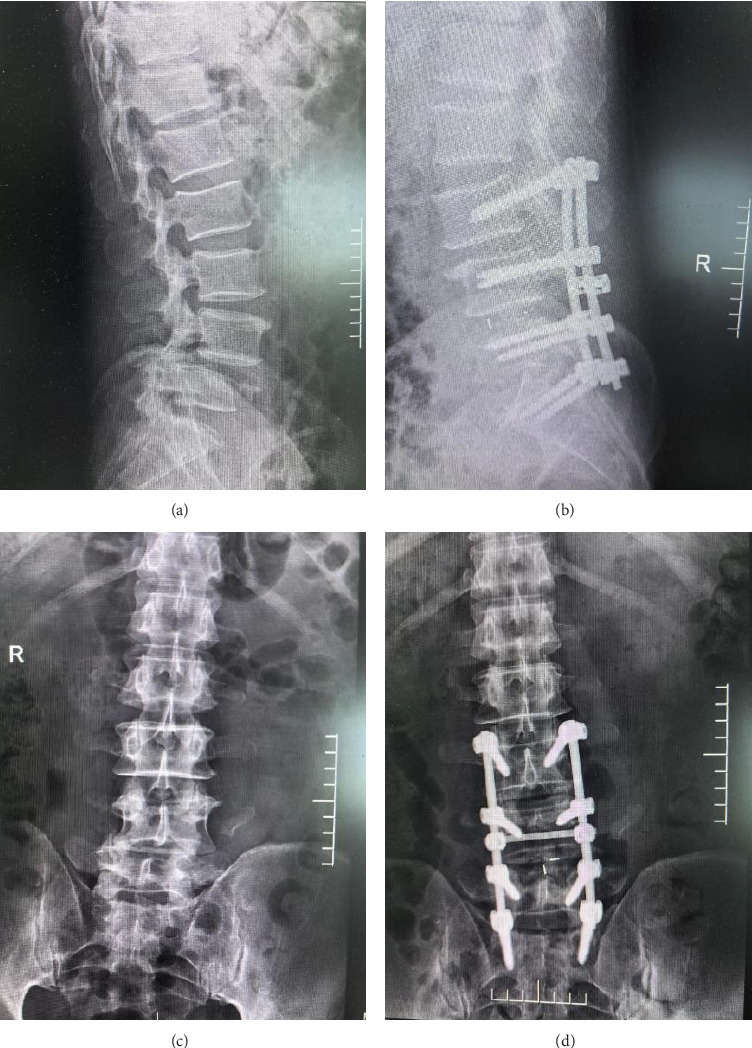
Radiographic images of the thoracolumbar spine in a patient with spinal cord injury before and after posterior decompression and internal fixation surgery: (a) preoperative lateral view; (b) postoperative lateral view; (c) preoperative anteroposterior view; (d) postoperative anteroposterior view.
